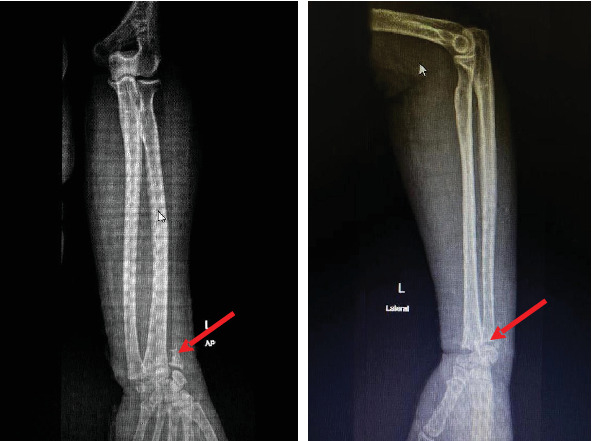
Plain X-ray of the left hand (AP and lateral) showing a left distal radial fracture with radiocarpal joint dislocation.
